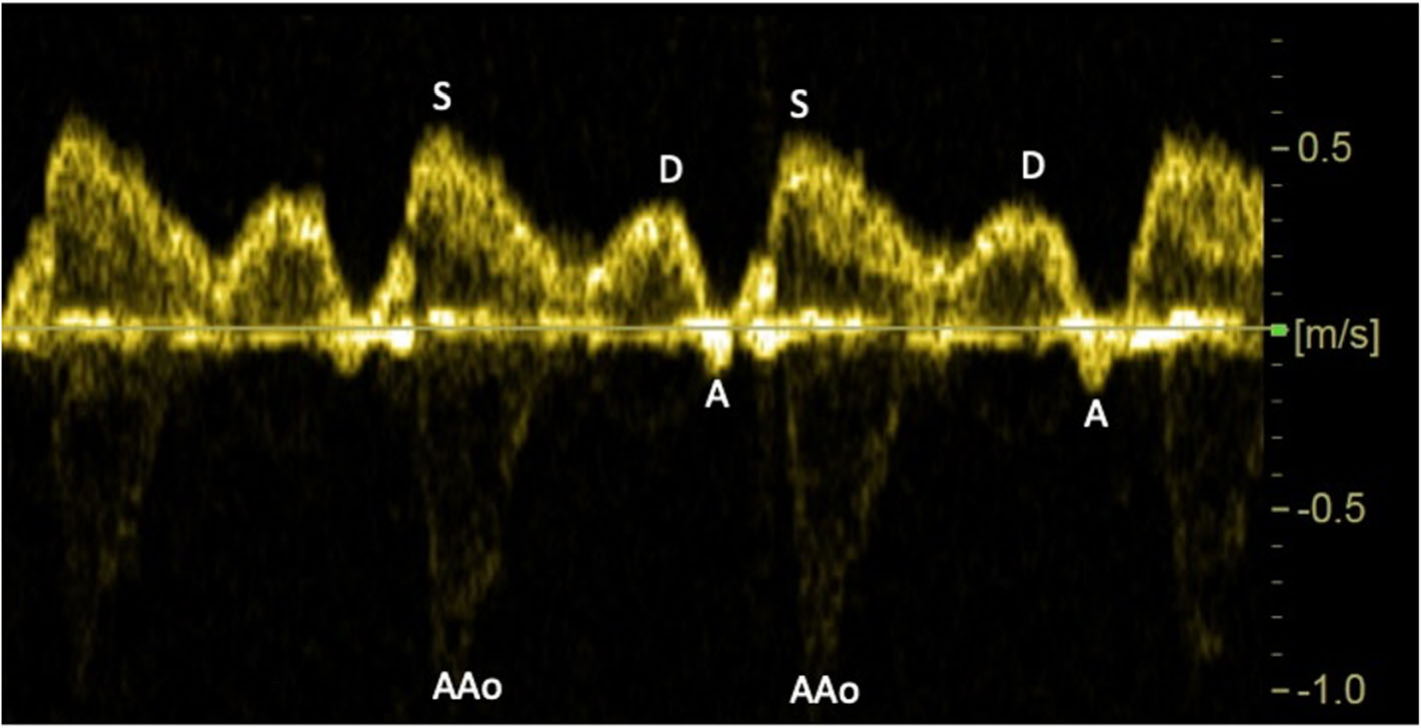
Doppler recording of the fetal superior vena cava blood velocity waveforms showing systolic peak velocity (S), diastolic peak velocity (D), and velocity deflection during atrial contraction (A). Interference by the systolic peak in the ascending aorta (AAo) seen below the zero line
